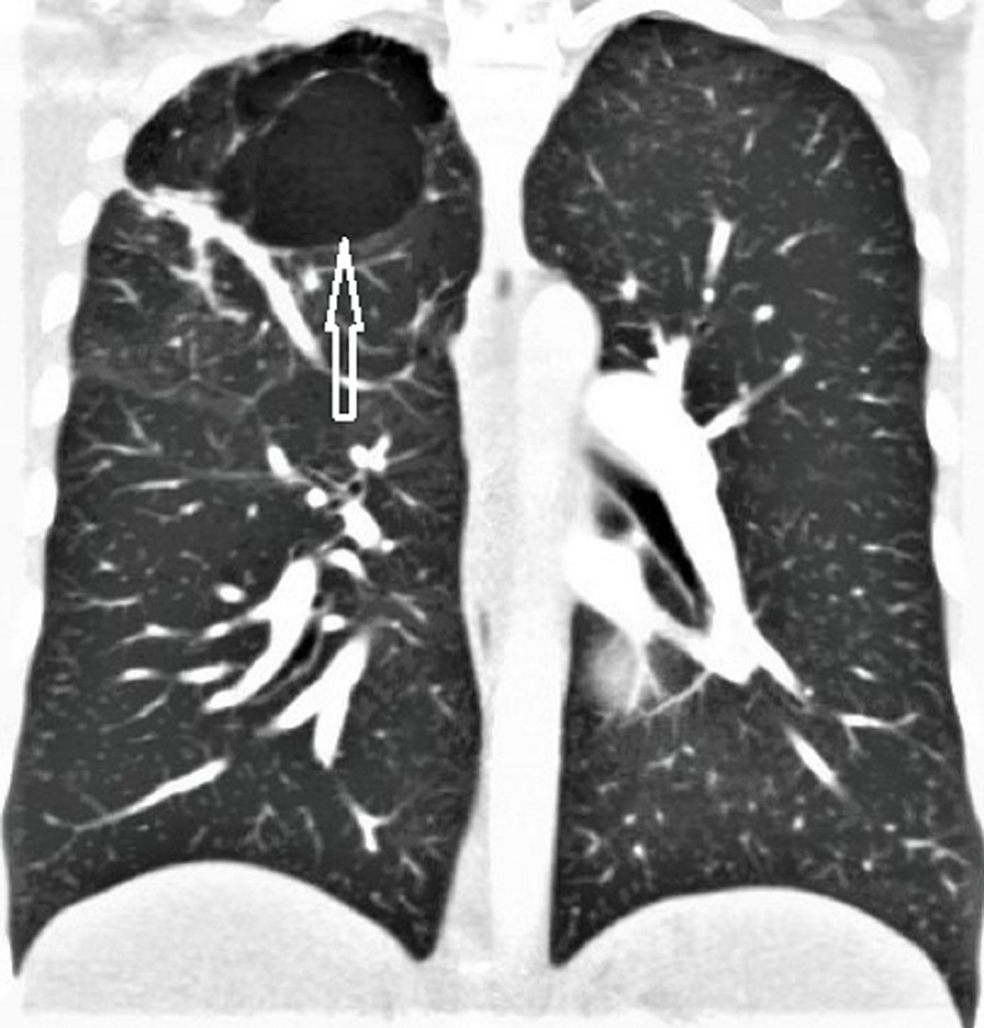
CTA showing one of multiple large bullae in the right upper lobe with associated pleuro-parenchymal scarring (arrow)
